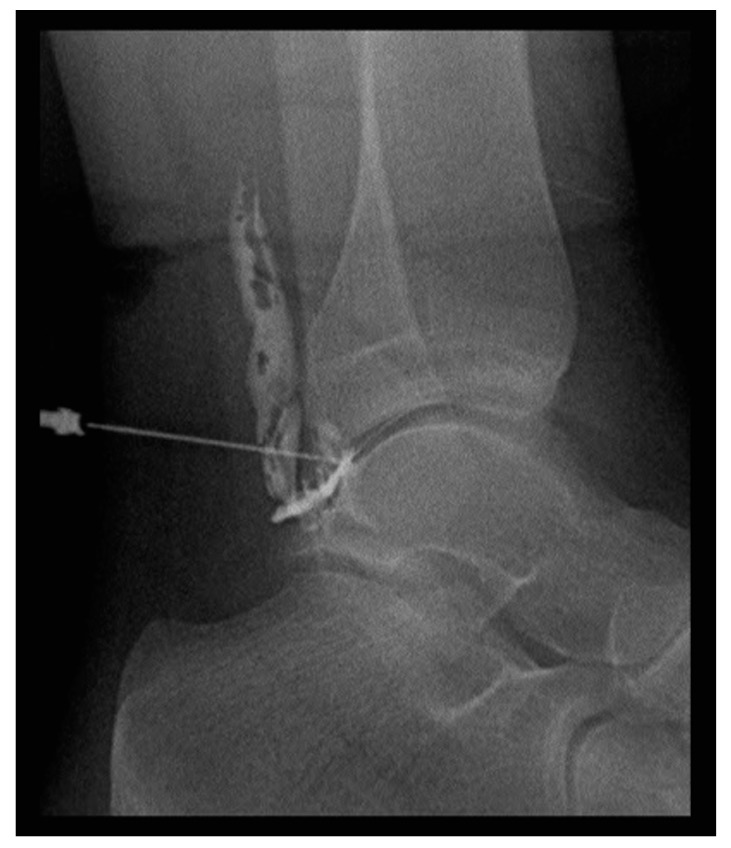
X-ray of the ankle joint confirming correct needle position before application of erbium-169 (^169^Er).
